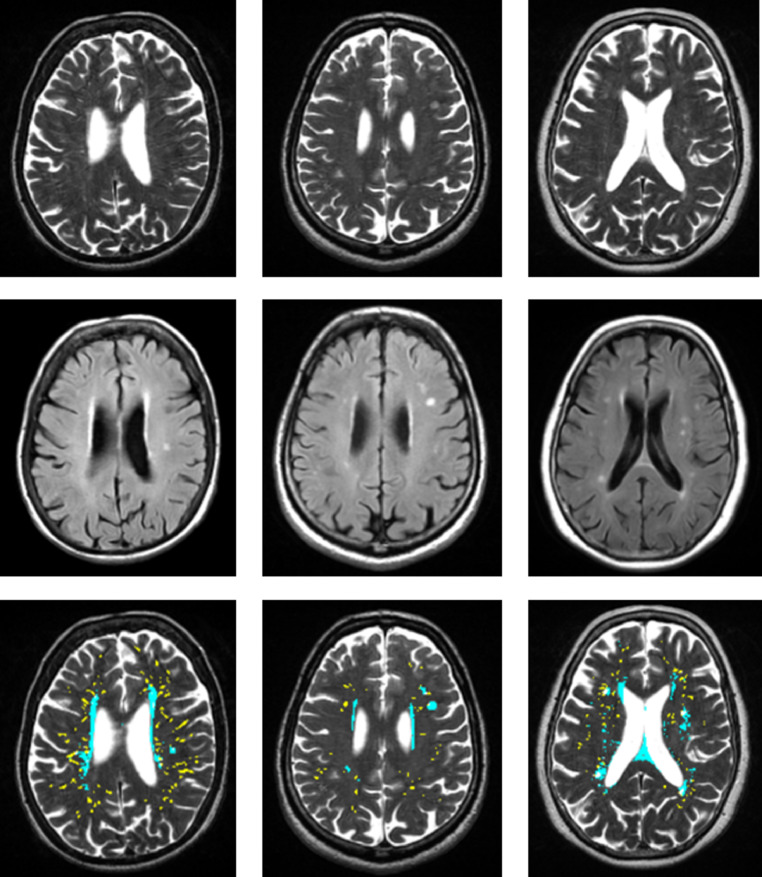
Bottom row: Examples of PVS (yellow) and WMH (cyan) segmentations. The middle and left images show WMH clearly separate from PVS. The right image shows some WMH and PVS overlap, however WMH are around PVS (the method did not segment all the WMH as PVS). Top and middle rows: Corresponding T2w and FLAIR source images. (For interpretation of the references to colour in this figure legend, the reader is referred to the web version of this article.)
